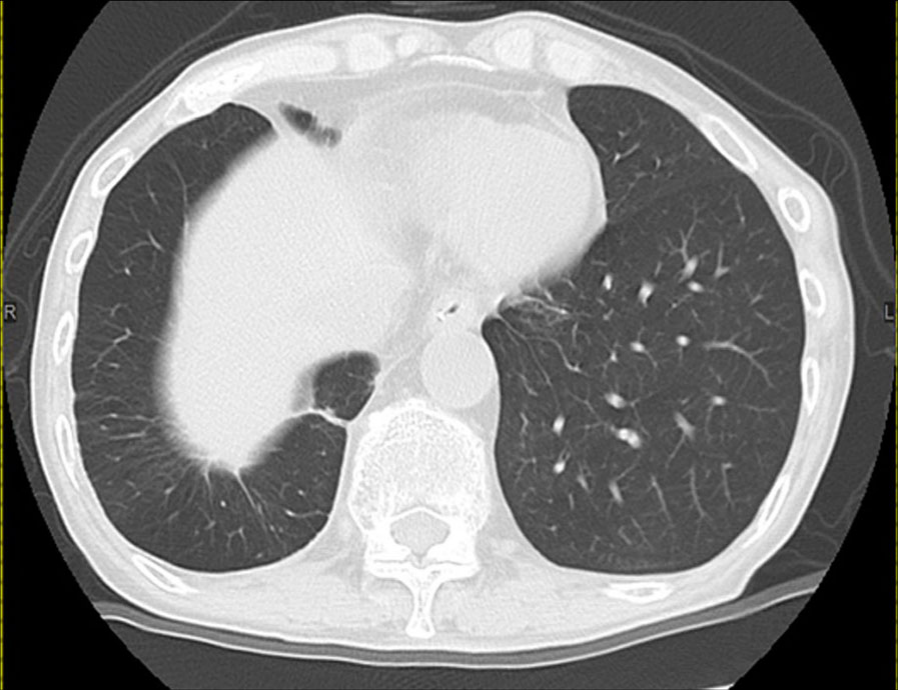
Image of chest CT 6 months after the treatment with antibiotics. The pulmonary actinomycosis disappeared
